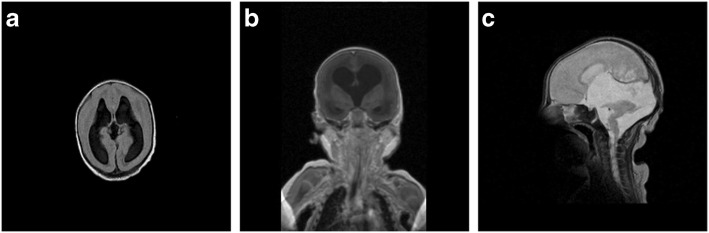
Cerebral MR-imaging of a patient with Walker-Warburg syndrome (WWS) at the age of 12 days (patient 21 a) showing bilateral enlargement of the internal ventricles and cobblestone lissencephaly (Type 2) with lack of gyration (a: axial fluid attenuated inversion recovery [FLAIR]; b: coronal magnetization-prepared rapid gradient echo [MP-RAGE]). The mid-sagittal section scan (c) demonstrates kinking of the brainstem and cerebellar dysplasia with absent vermis. Note the small corpus callosum (T2 weighted turbo spin echo [TSE])
